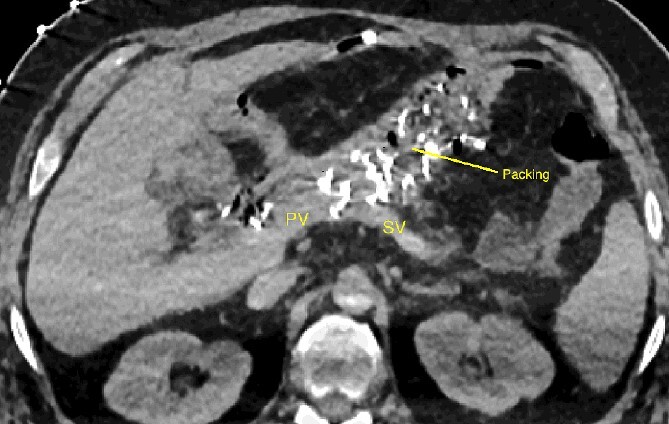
CT scan after relaparotomy located the packs used for hemorrhage control anterior to the confluence of the portal vein and splenic vein. PV portal vein, SV splenic vein.
